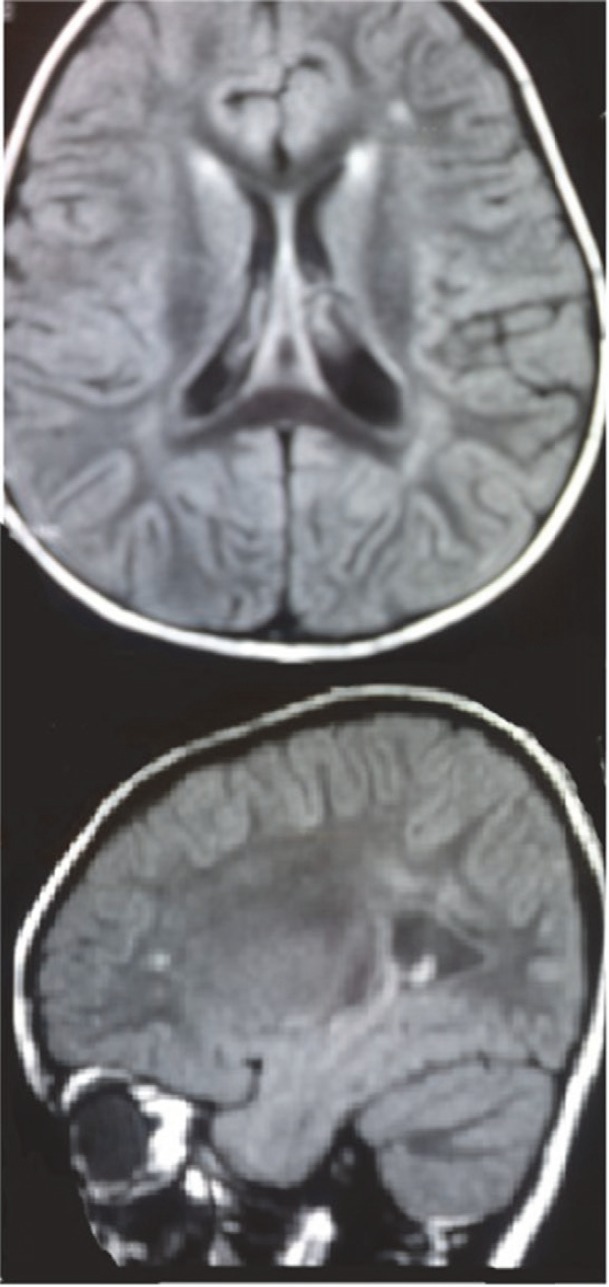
Round hyperintense frontal subcortical lesion in brain MRI
